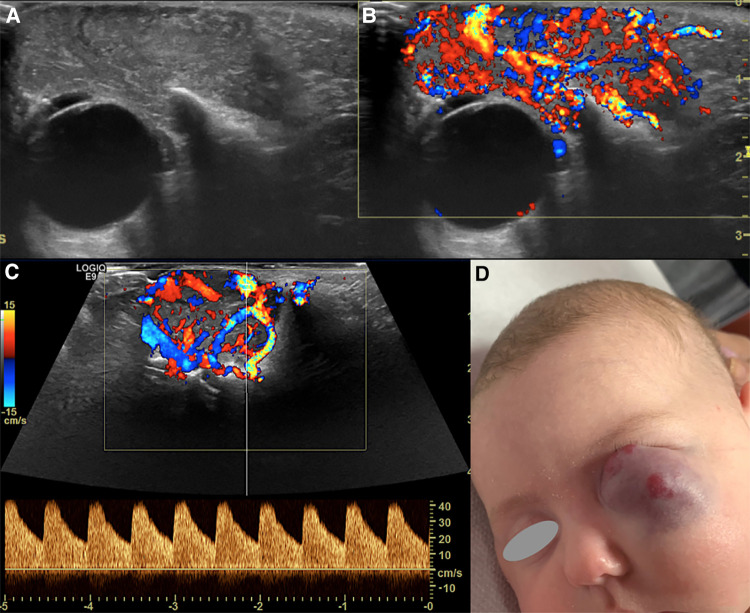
Us of an infantile hemangioma (IH) of the left orbit in a 5-month-old boy, during the proliferative phase. B-mode US shows a hyperechoic mass with well-defined margins overlying the globe (A) and a very high vascular density at Color-Doppler US (B). Spectral analysis revealed the presence of low resistance arterial flow (C). The clinical appearance of the lesion is depicted in (D).
